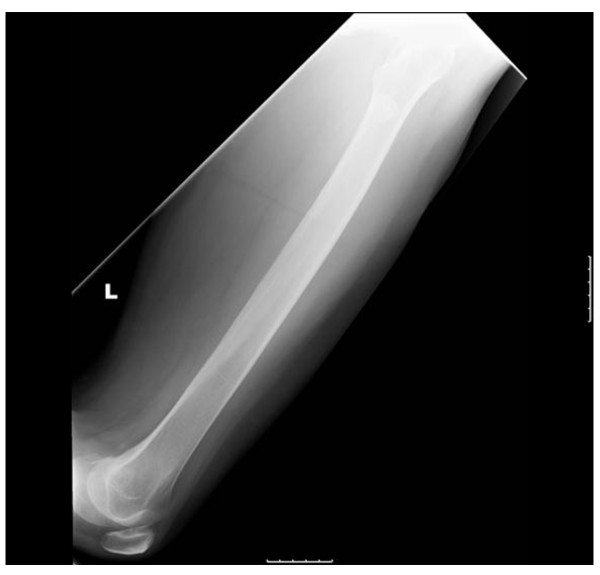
Anteroposterior radiographs of the left distal femur six months after diagnosis with resolution of symptoms.
